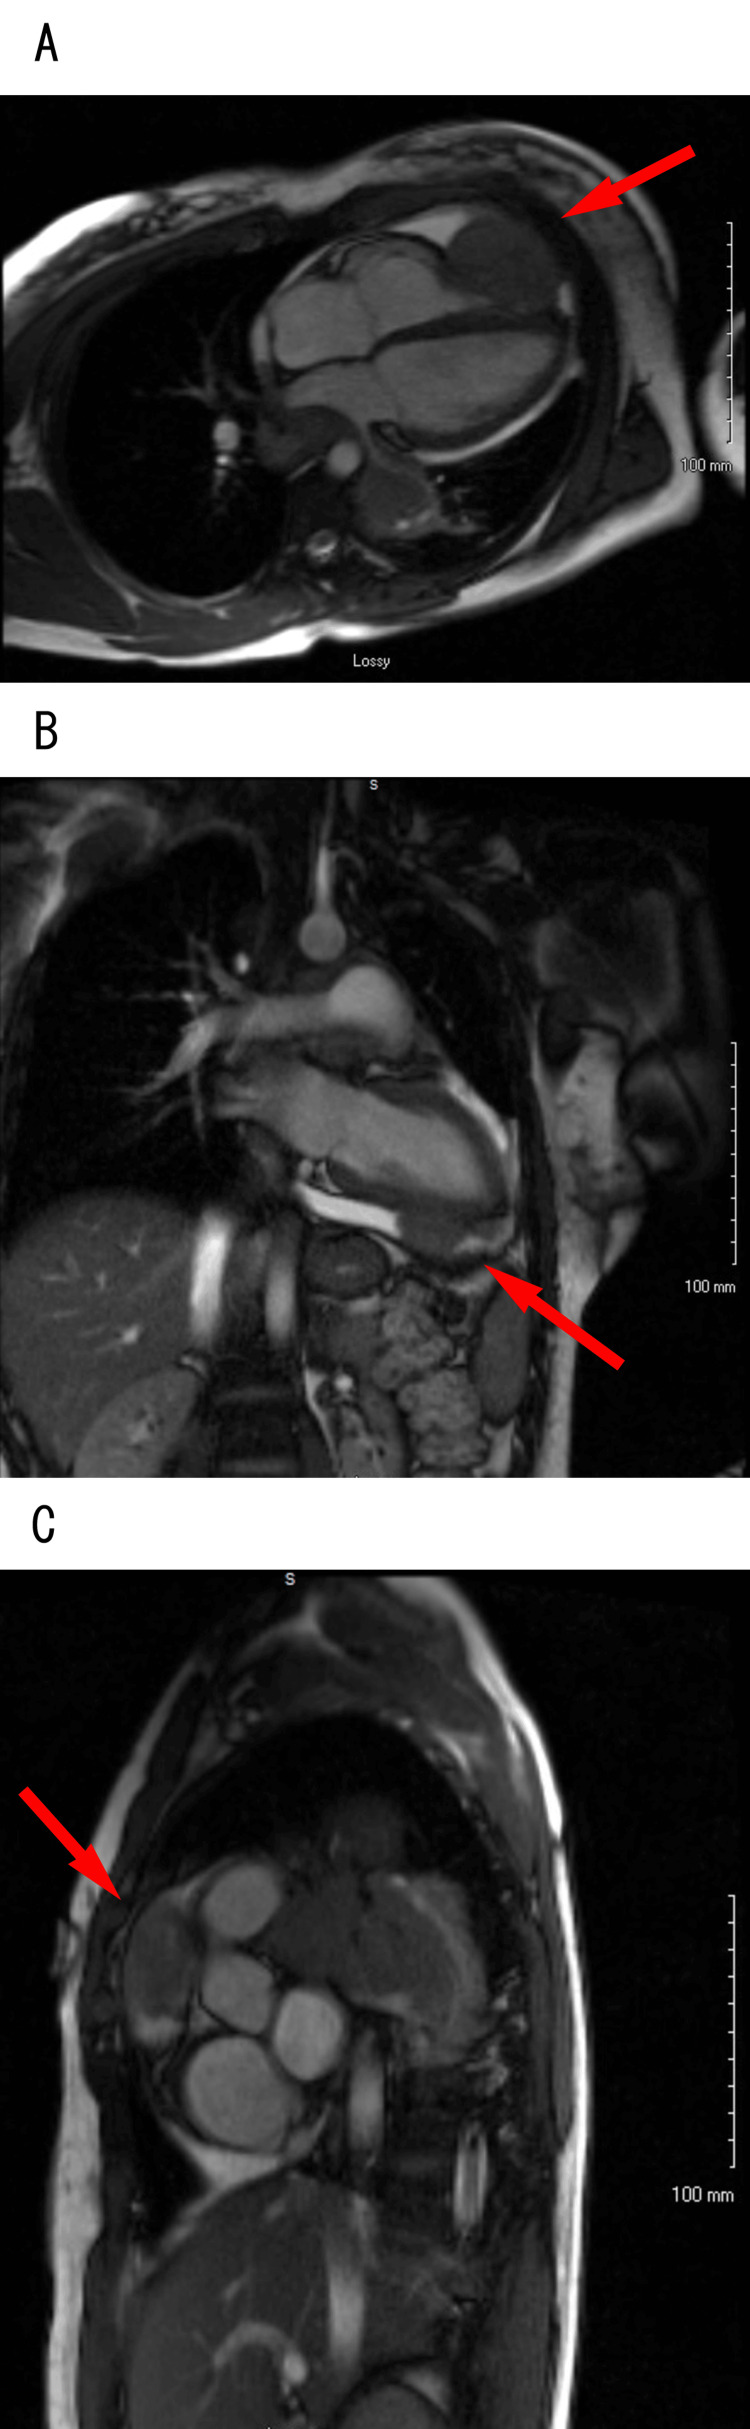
Cardiac magnetic resonance imaging Magnetic resonance imaging cine longitudinal relaxation time (T1) imaging of the (A) four-chamber view and (B) two-chamber view showed a 6 cm x 5 cm intrapericardial mass adjacent to the right ventricle apex without clear demarcation between the right ventricle free wall and mass. (C) Magnetic resonance imaging cine longitudinal relaxation time (T1) imaging of the short axis showed an intrapericardial mass anterior to the right ventricle outflow tract/pulmonary artery.
